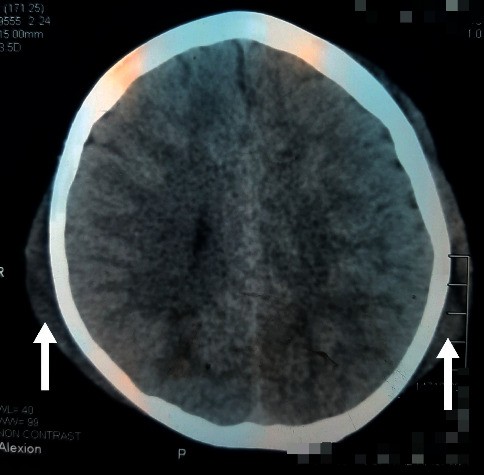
Noncontrast CT scan showing the soft tissue swelling of the scalp (arrows).
